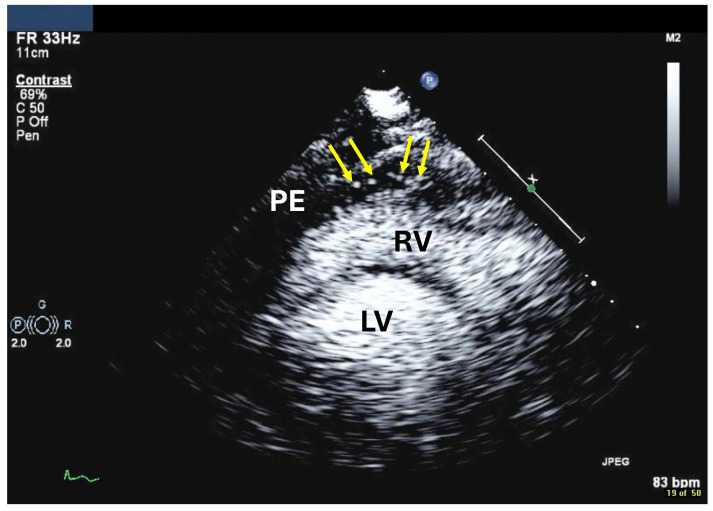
Contrast bubbles in the pericardial space (arrow) in a patient with bleeding into the pericardium, modified parasternal long-axis view, bubbles (arrows) are displayed anteriorly to the RV in the pericardial effusion (PE). NOTE: often only isolated contrast signals are displayed in the pericardial effusion. RV right ventricle, LV left ventricle.
